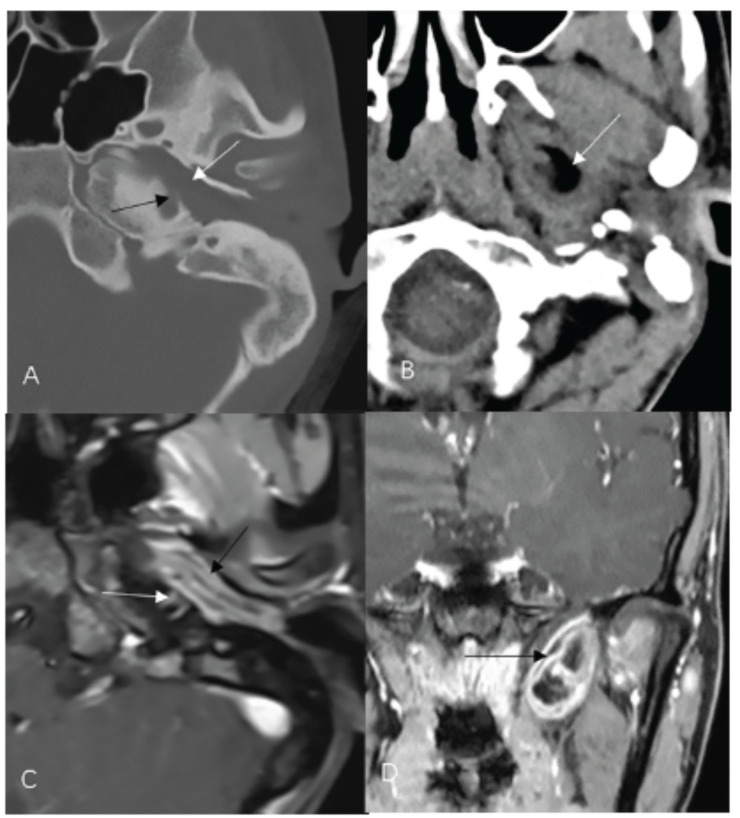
MET involved EAC, tympanum, ET and parapharyngeal spaces, patient No. 3. (A), axial CT Bony algorithms reconstruction imaging, internal carotid artery canal slightly destruction (black arrow), ET expanded (white arrow). (B), axial CT soft tissue algorithms reconstruction imaging, MET involved parapharyngeal space (white arrow), with thick-walled capsules. (C), axial fat-suppressed T1WI enhancement. Capsules had two layers of soft tissue sandwiched by a thin layer of adipose tissue (black arrow), internal carotid artery (white arrow). (D), coronal fat-suppressed T1WI enhancement. Part of the MET in the parapharyngeal space, thick-walled capsules with sandwich sign (black arrow), and central soft and capsule enhanced.
